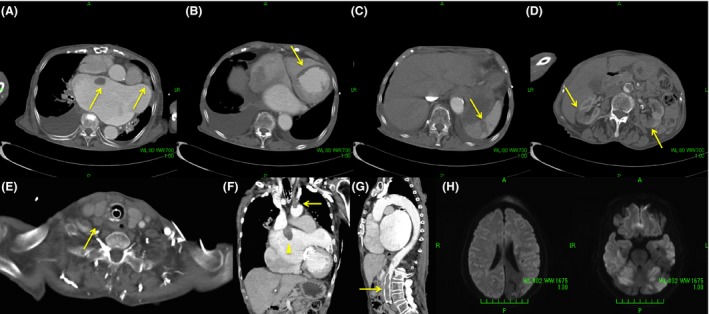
Contrast computed tomography scan and diffusion‐weighted image of magnetic resonance imaging in an 82‐year‐old woman with atrial fibrillation. A, Thrombi in the left atrium (arrows). B, Poor contrast in the left ventricle (arrow). C, Poor contrast in the spleen (arrow). D, Poor contrast in bilateral kidneys (arrows). E, Contrast defect in the right common carotid artery (arrow). F, Thrombus at the bifurcations of the left common carotid artery and subclavian artery (arrow) and thrombus in the left atrium (arrow head). G, Occlusion of the aorta (arrow). H, Diffuse high‐intensity area in the cerebrum and cerebellum.
